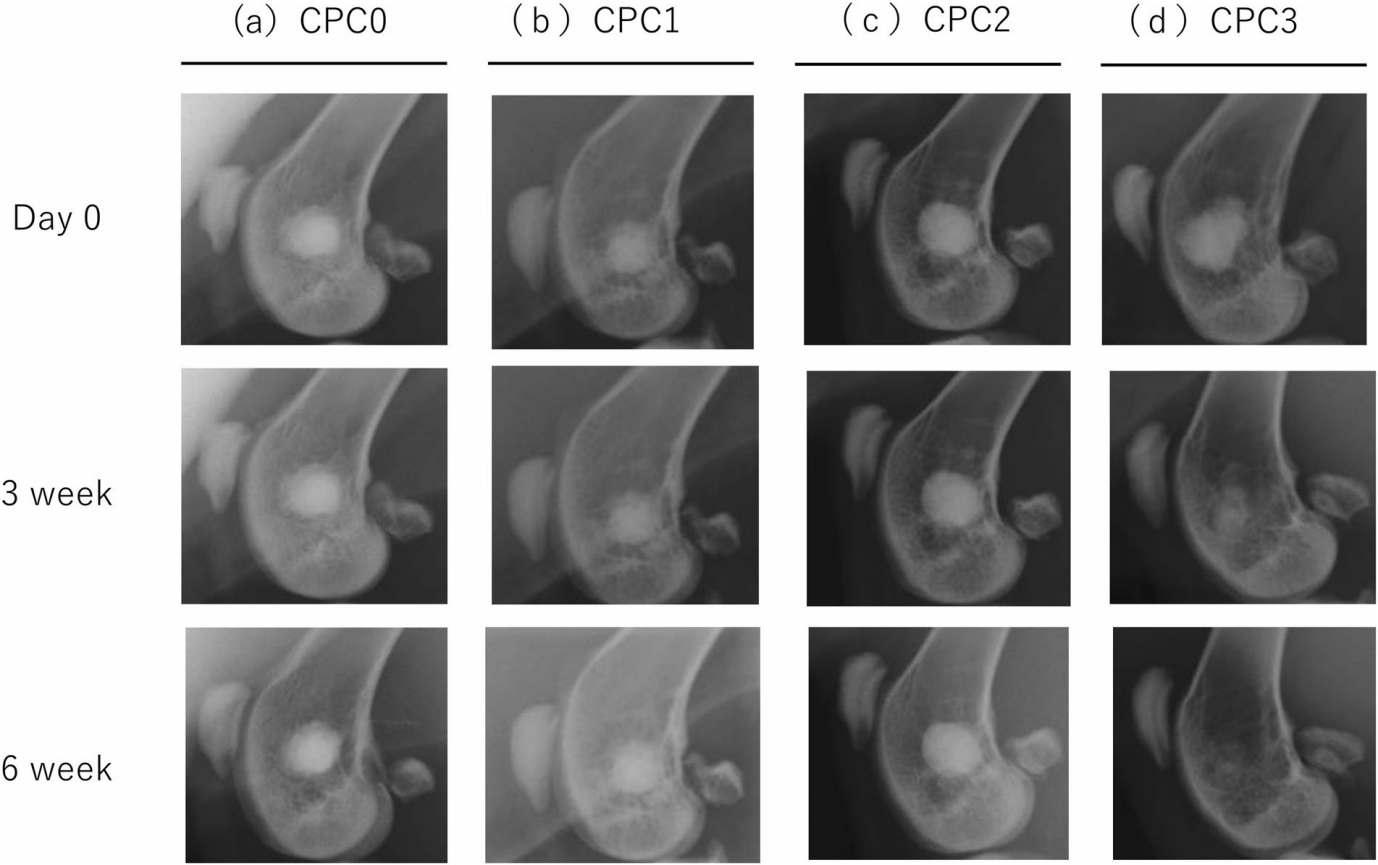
X-ray images of CPCs at 0 day, 3 weeks, and 6 weeks after surgery. Impaired portion of femoral condyle indicates the CPCs. Only CPC3 displays a time-dependent increase in permeability at the site. This is especially noticeable in the image after 6 weeks
